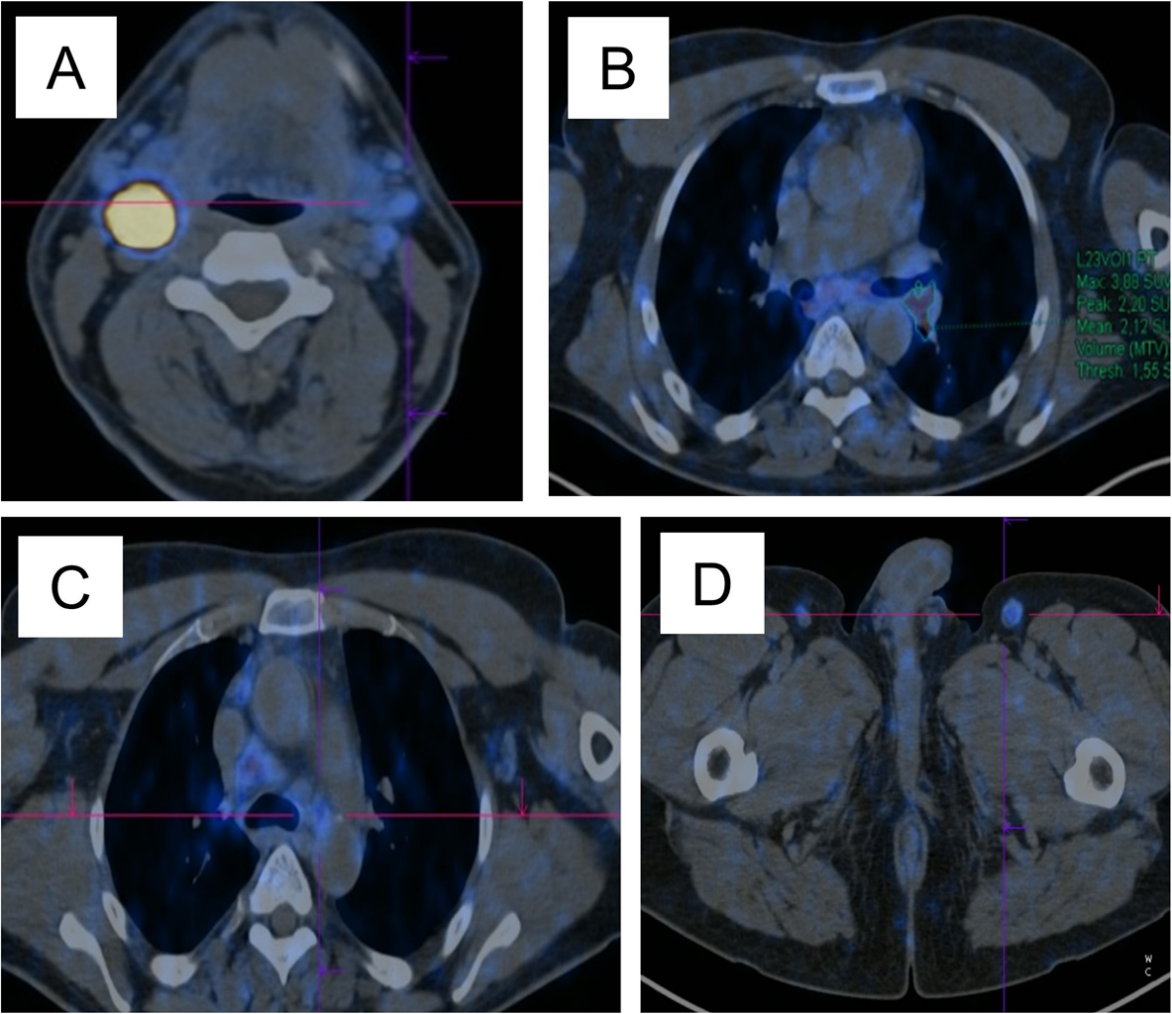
1,4,7,10-tetraazacyclododecane-N(I),N(II),N(III),N(IIII)-tetraacetic acid(D)-Phe(1)-thy(3)-octreotide (^68^Ga-DOTATOC) positron emission tomography/computed tomography axial fused images findings. a Intense focal uptake (maximum standardized uptake value 92.4) in the neck mass, strongly suggestive of paraganglioma; b hilar-mediastinal and peribronchial weak uptake (maximum standardized uptake value 3.9 in the pulmonary left hilum); c axillary and d inguinal weak uptake
